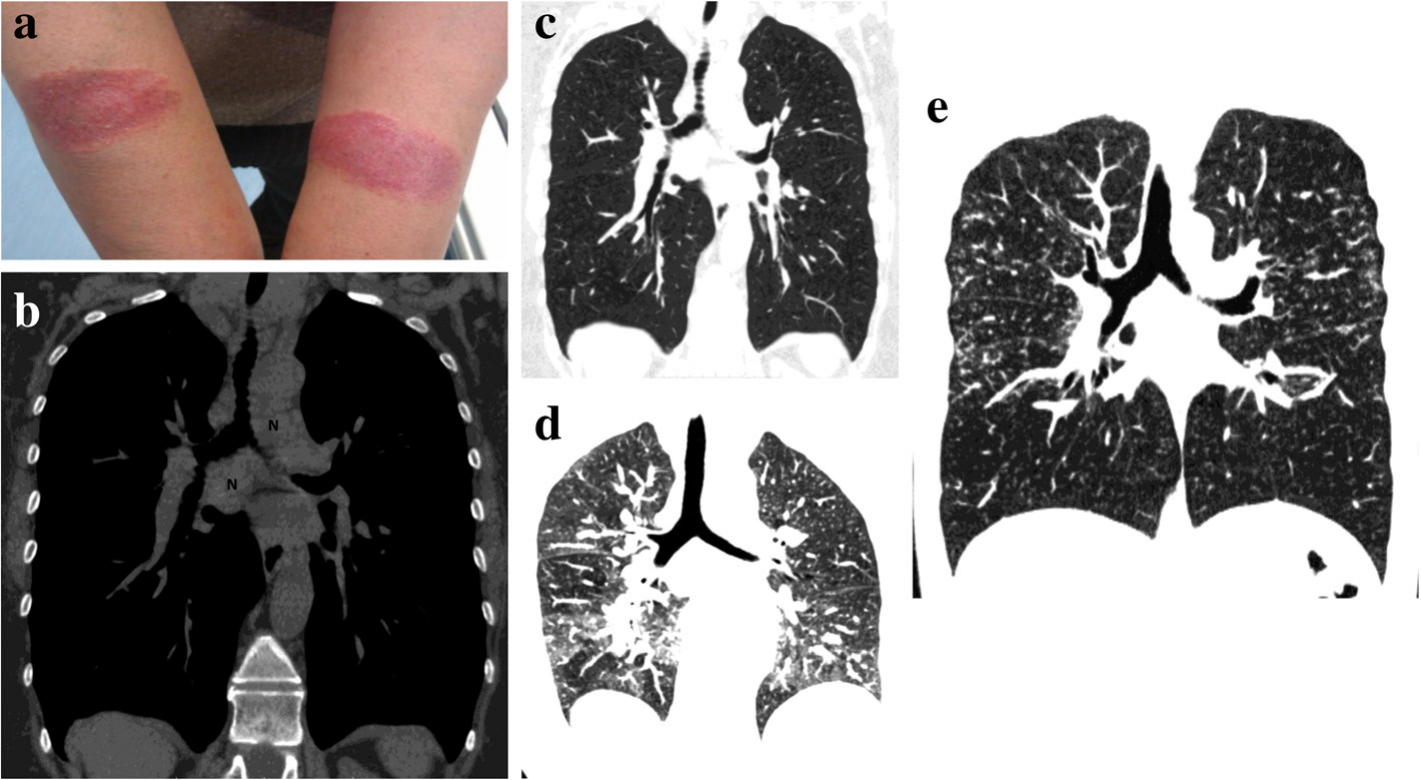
38-year-old woman with cutaneous and pulmonary sarcoidosis complaining of a slight exertional dyspnea. Photograph of volar forearms shows red-to-purple indurated plaques. Biopsy demonstrated cutaneous sarcoidosis (Lupus Pernio) (a). Coronally-reformatted inspiratory CT image with soft tissue window settings, obtained two weeks after cutaneous biopsy, demonstrates mediastinal lymphoadenopathies (N) (b). Coronally-reformatted inspiratory CT image with lung settings through the same level of (b) shows absence of lung abnormalities (c). Extensive air-trapping can be seen on coronally-reformatted expiratory CT image (d). Coronally-reformatted inspiratory CT image, obtained six months after, shows typical sarcoidosis lung pattern (multiple micronodules with a perilymphatic distribution both in lower and upper lobes) (e).
